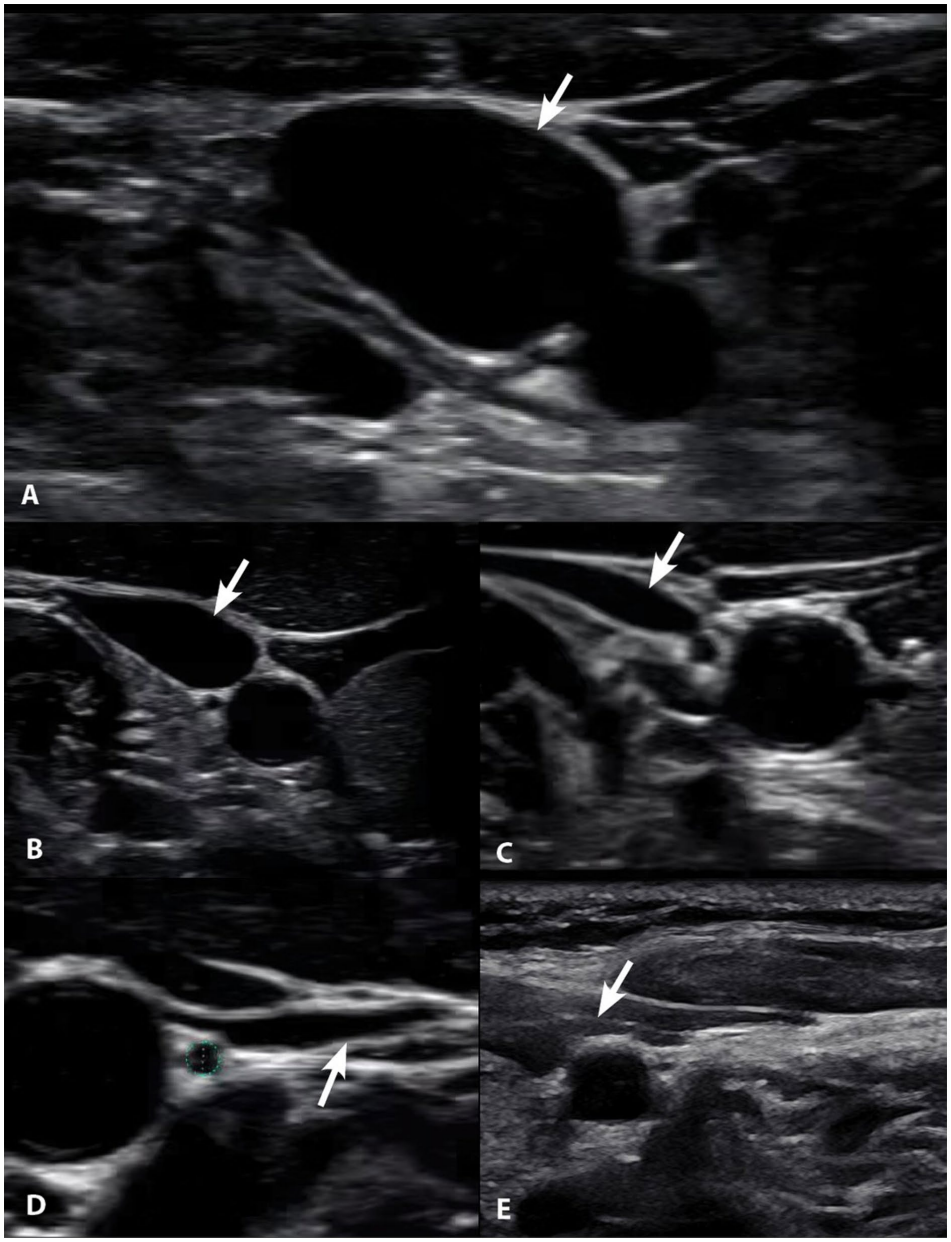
Degrees of internal jugular vein compression as seen on ultrasound examination of the neck. (A) Normal, “open” internal jugular vein. (B) Slightly compressed. (C) Moderately compressed. (D) Severely compressed. (E) Completely closed. It is internal jugular vein compression (arrows), especially with upright posture and neck motions, that leads to intracranial hypertension (increased brain pressure).
